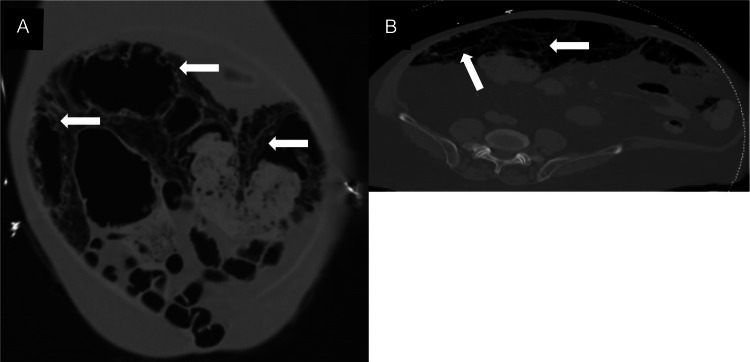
54-year-old with history of Crohn’s disease and disseminated Mycobacterium Avium Complex (MAC) on long-term steroids, presents with back and abdominal pain with nausea. Coronal (A) and axial (B) CT images show PI of the colon with cystic and linear patterns (arrows) and extensive air throughout the mesentery. Workup revealed mild tenderness to palpation, normal WBC and lactate levels. The patient was treated conservatively with bowel rest, IV fluids, and antibiotics
